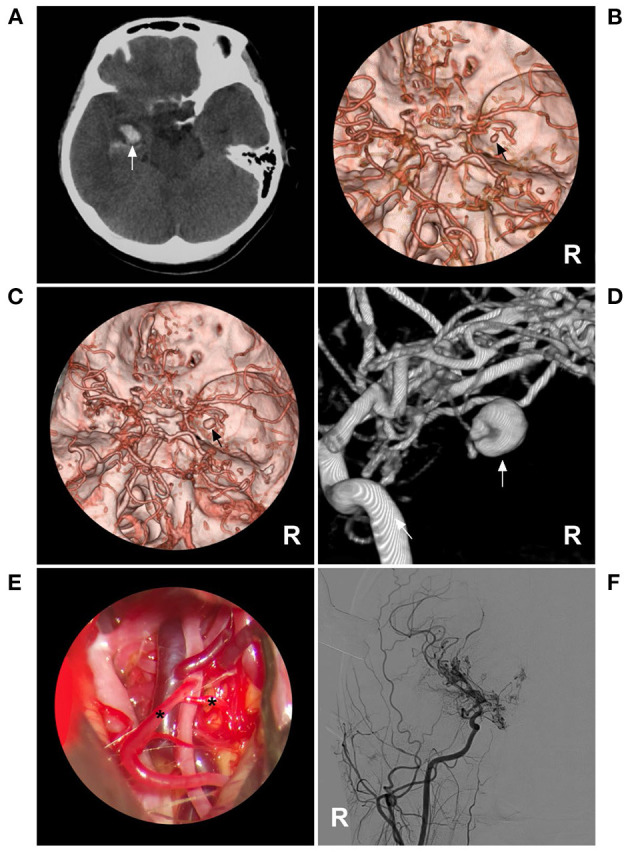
A growing aneurysm in case 2 in Table 2. (A) CT showed hemorrhage (arrow) in the right temporal lobe involving the ventricle. (B) CTA showed an aneurysm (arrow) in the right moyamoya vessels, and bilateral MMD can be seen. (C) One week later, repeated CTA showed that the aneurysm had grown (arrow). (D) Three-dimensional DSA of the right ICA showed that the aneurysm (arrow) vessels affected by MMD were enlarged. (E) Intraoperative image showing the vessels affected by MMD (asterisks); these vessels appeared thin and frail. (F) Postoperative DSA after aneurysm resection showed that the aneurysm had disappeared. CT, computed tomography; CTA, computed tomography angiography; DSA, digital subtracted angiography; ICA, internal carotid artery; MMD, moyamoya disease; R, right.
